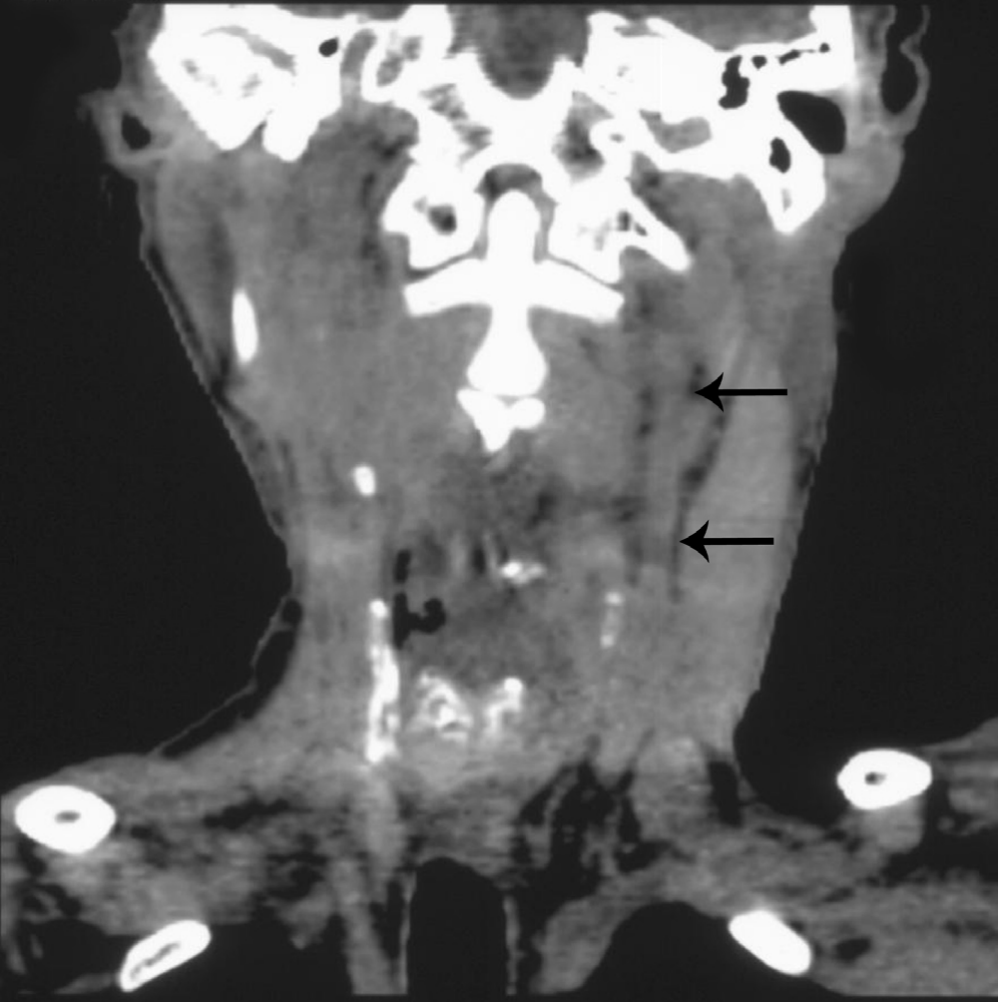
CT frontal reformation of the neck extracted from the 18 FDG-PET/CT imaging. The arrows show the reconstructed carotid artery.
